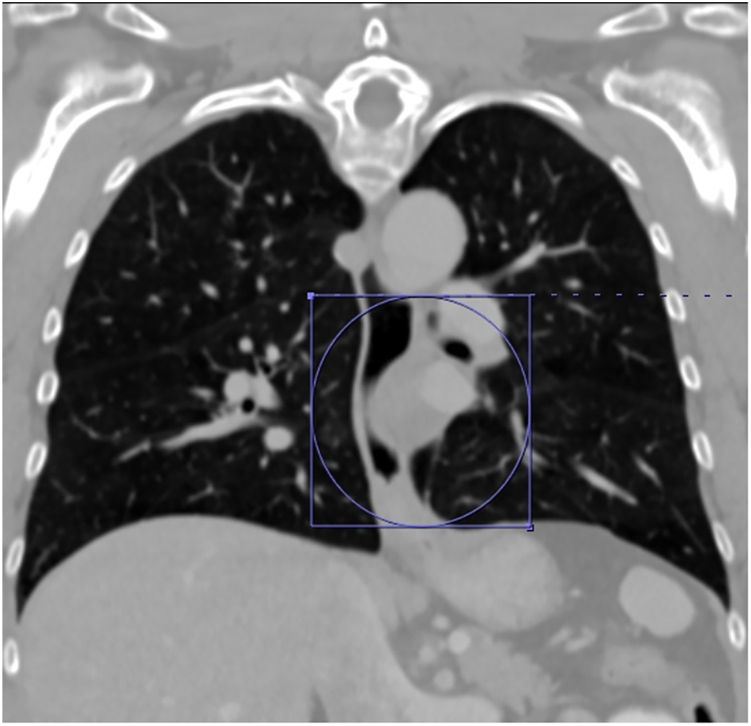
Computed tomography of the chest with intravenous administration of contrast material demonstrating a smoothly marginated 2.6 × 2.7-cm paraesophageal mass to the left of the midesophagus with extrinsic compression of the esophagus (mass shown within circle).
